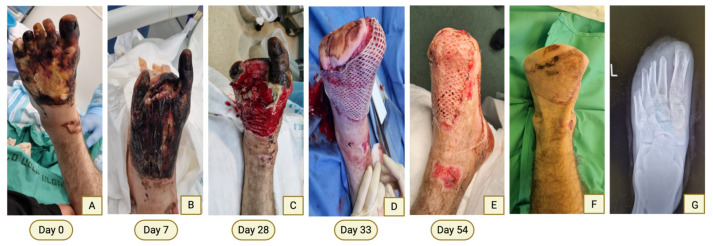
Clinical course following electrical injury to the left foot. (A) Extensive necrosis involving the dorsal foot and toes two weeks post injury. COD treatment was initiated. (B) Evolution to eschar formation with subsequent amputation of middle toes. (C) After three additional weeks of COD therapy: progressive autolytic debridement with replacement by dense, well-vascularized granulation tissue. (D) Definitive surgical management: trans-metatarsal amputation with preservation of plantar skin and split-thickness skin grafting onto a well-prepared wound bed. (E,F) Complete graft taken two weeks and two months post grafting. (G) Corresponding radiographic image.
